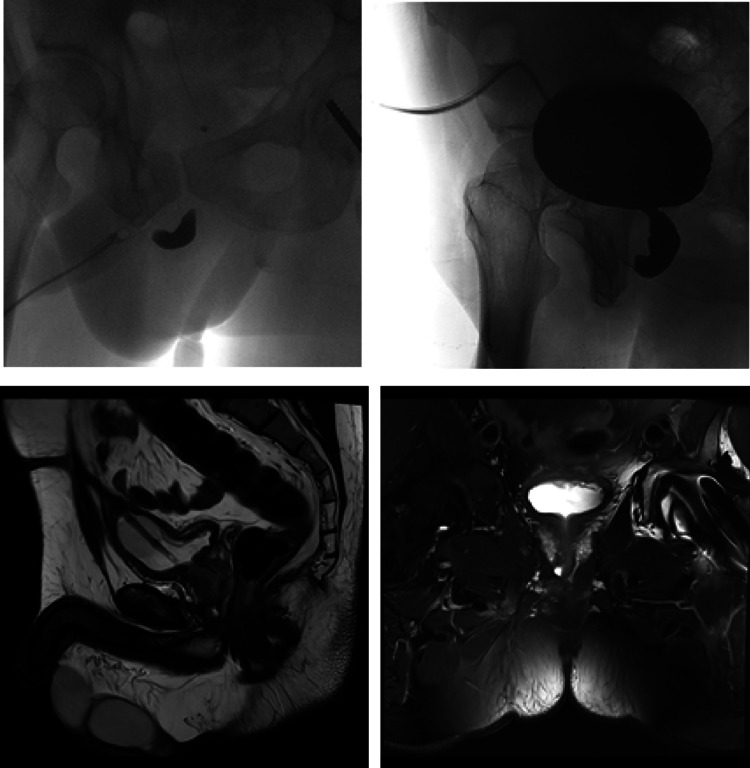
Preoperative retrograde urethrography, voiding cystourethrography, and MRI
